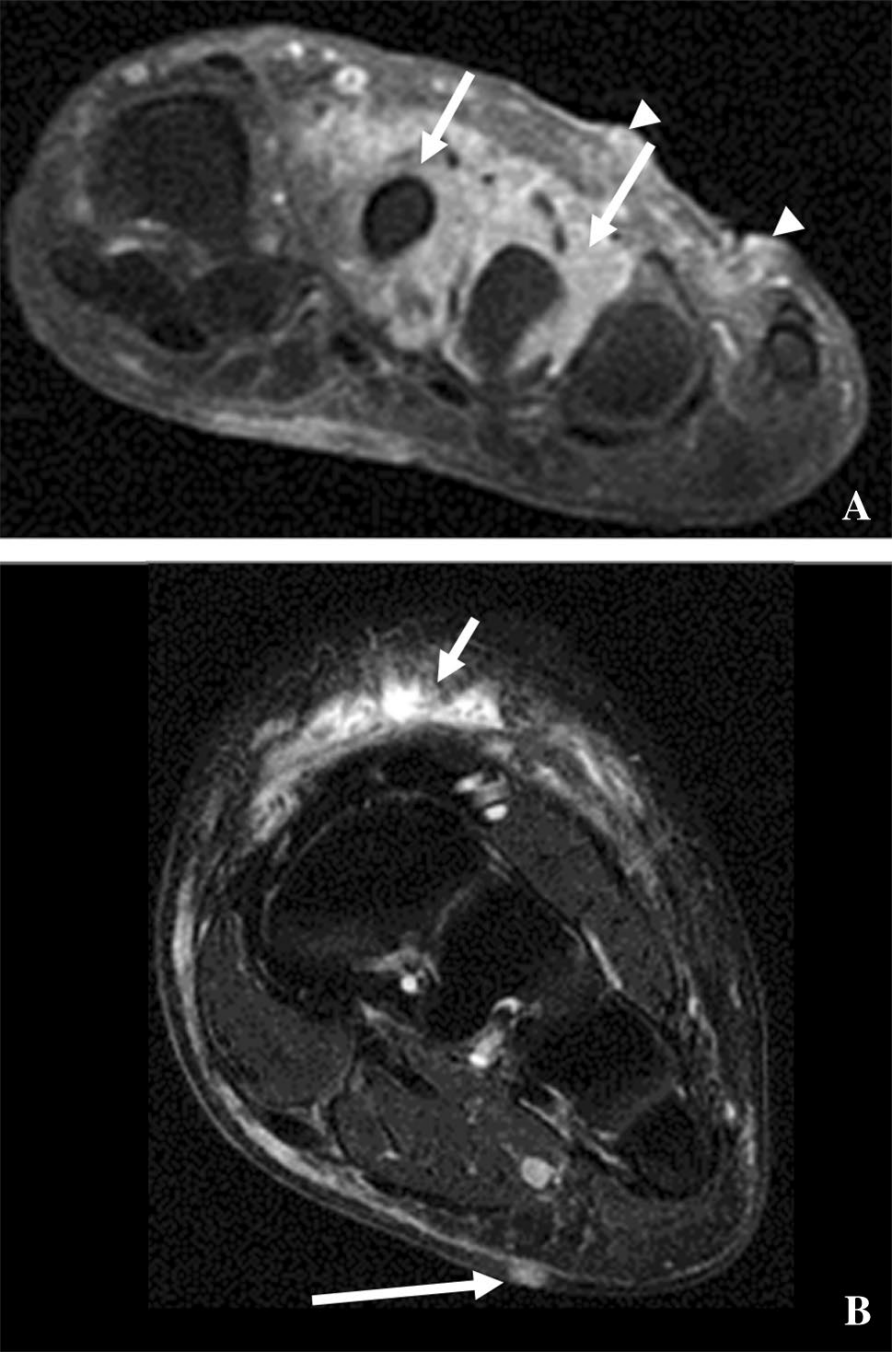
A A 67-year-old man with KS. MRI of left foot. Contrast-enhanced, fat-suppressed axial image showing thickened and enhancing soft tissue (arrows) surrounding the second and third metatarsal and enhancing skin nodules (arrowheads). B A 45-year-old man with KS. MRI lower extremity T2 fat-saturated axial image with heterogeneously hyperintense subcutaneous tissue (short arrow) and a midline plantar nodule (long arrow), both of which are consistent with KS
